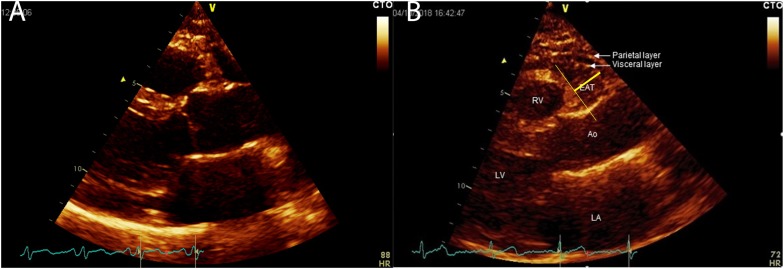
EAT thickness visualization and measurement in parasternal long axis view; panel (A) thin epicardial adipose tissue; panel (B) thick epicardial adipose tissue. EAT, Epicardial Adipose Tissue; LA, left atrium; LV, left ventricle; Ao, ascending aorta; RV, right ventricle. EAT is visualized between the visceral layer of the pericardium an the myocardium at the Rindfleisch fold.
